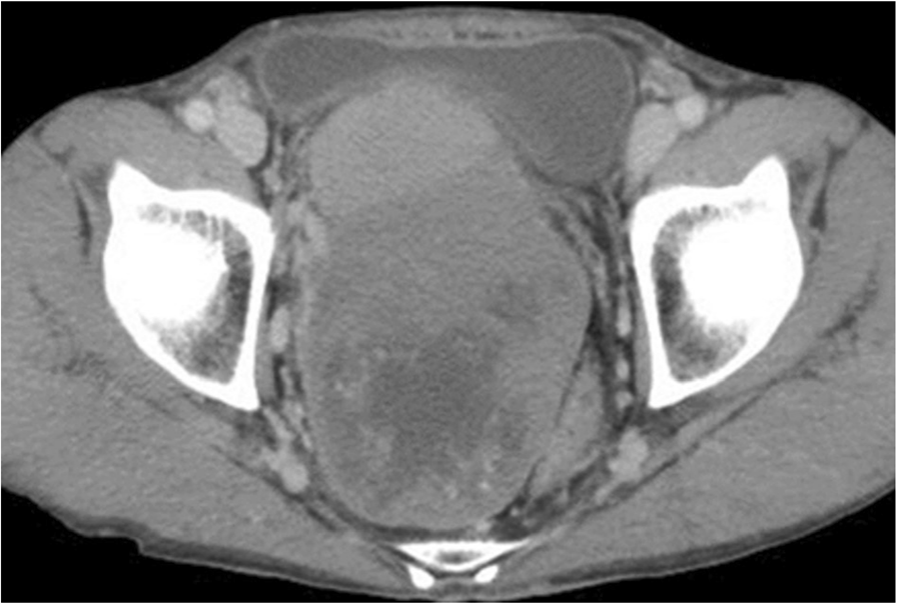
Contrast-enhanced CT image showing a mass occupying the pelvic cavity. The tumor was heterogeneously enhanced
